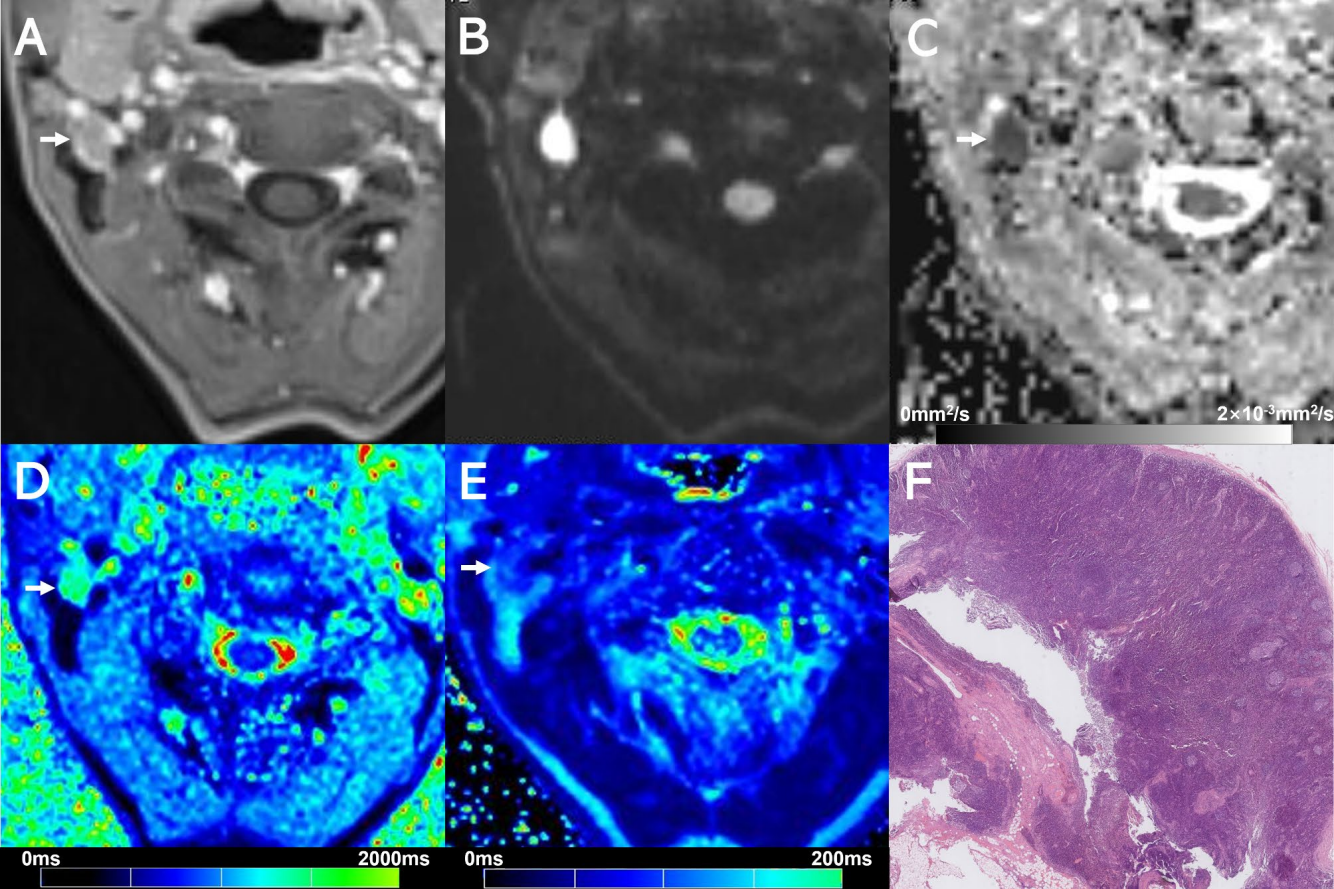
Images show a 7.9-mm size benign lymph node (arrows) at right level II from a 49-year-old male with hypopharyngeal cancer (the same patients in Fig. 2). (A) Contrast-enhanced T1-weighted image shows the lymph node (arrow). (B) DWI with a b-value of 800 mm^2^/s shows a hyperintense area. (C) The ADC map on the targeted lymph node shows a hypointense signal, an ADC value of (0.698 × 10^− 3^mm^2^/s). (D) T1 mapping and (E) T2 mapping show a T1 value of 1746.1 ± 115.1 ms and a T2 value of 92.0 ± 9.7 ms. (F) Section through the same lymph node showing absence of metastasis
